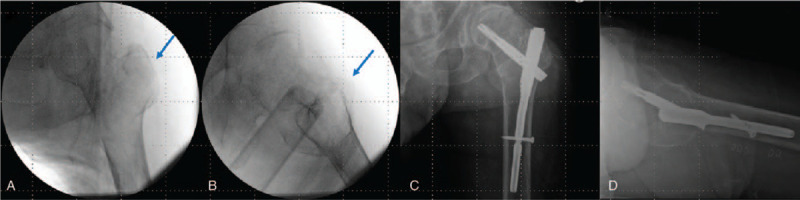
Case 2. (A) and (B) Intraoperative C-arm images showed that despite proper traction, complete reduction was not achieved and anterior angulation remained (thin blue arrow). (C) Postoperative radiographs showing adequate fracture reduction and proper fixation. (D) True lateral radiographs showing an apposition of the anterior cortex.
